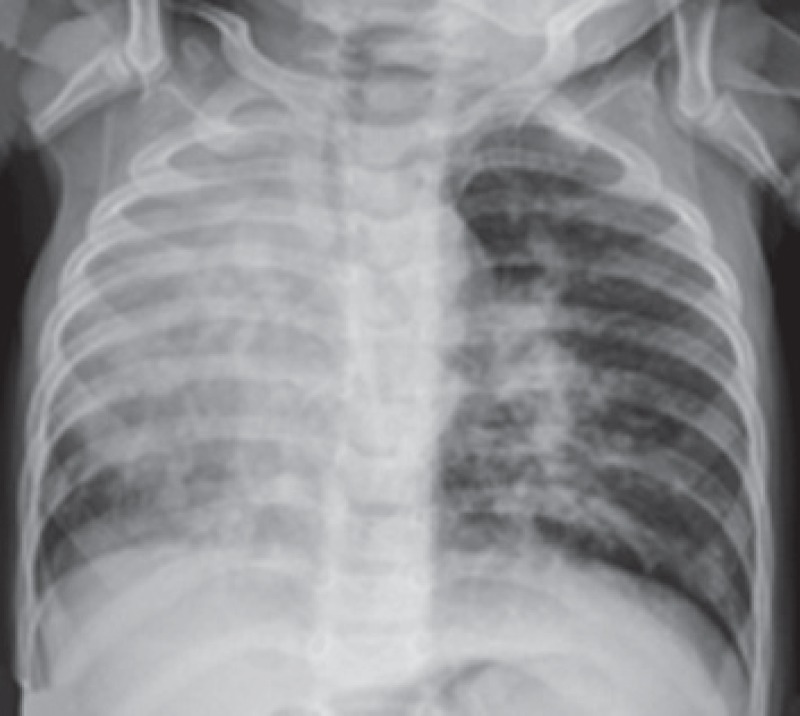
Chest X-ray showing cardiac dextroposition and radiopaque right hemithorax.
